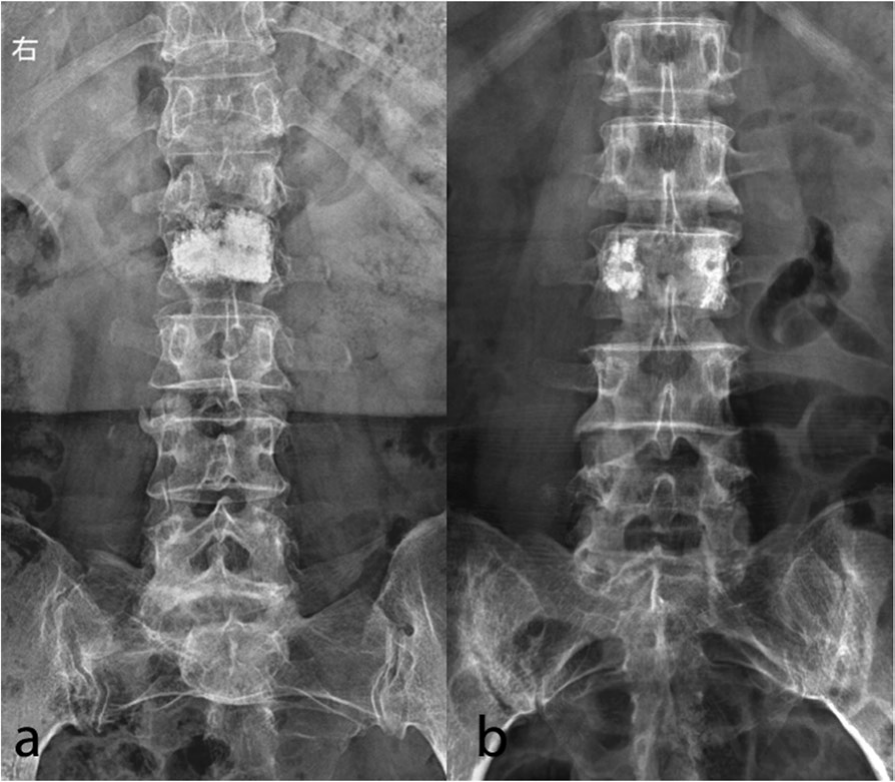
Postoperative frontal radiographs of the two bone cement distribution patterns. a Confluent bone cement distribution patterns. b Separated bone cement distribution patterns
